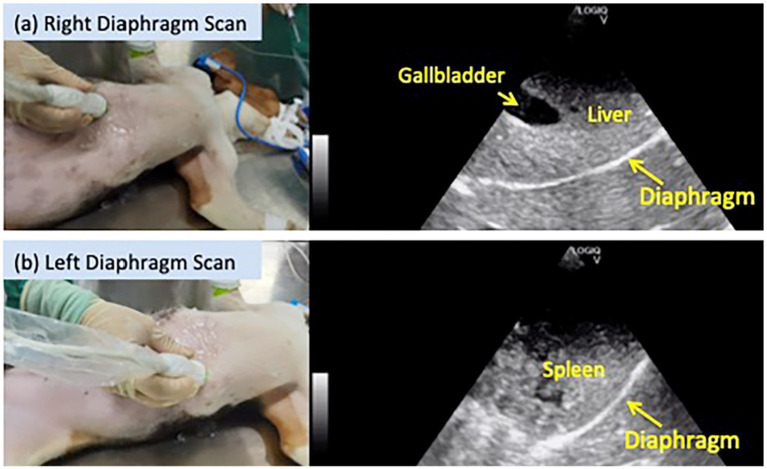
Ultrasound sections of the right and left diaphragm obtained with a portable ultrasound machine from beagle. (a) Right diaphragm of beagle; (b) Left diaphragm of beagle.
